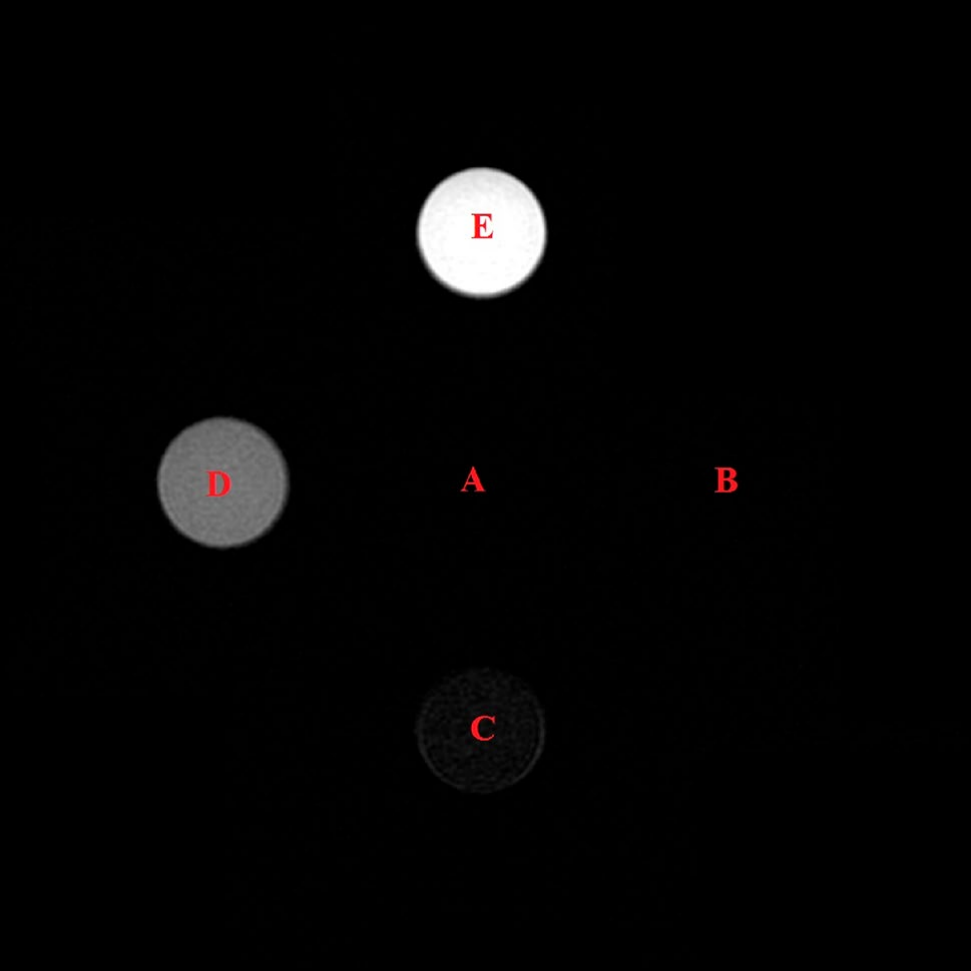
Magnetic resonance imaging evaluation (SSFSE TE: 1200 ms; TR 4000 ms) of five pure solutions with different Mn^2+^ concentrations.
